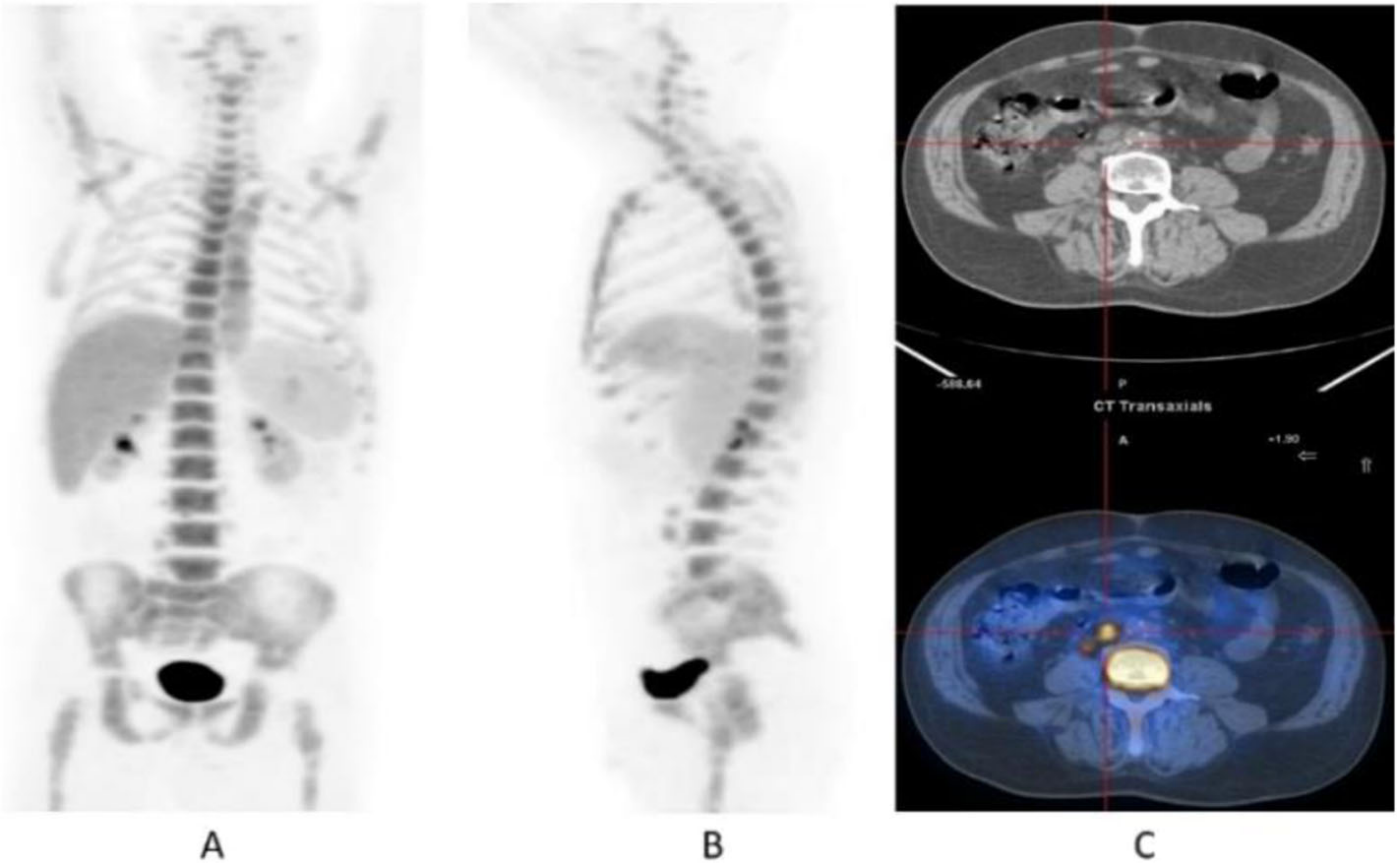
^18^F-FLT, Hodgkin lymphoma, response evaluation. *Clinical history*: 32 y.o. patient with Hodgkin lymphoma after second-line therapy. *PET/CT findings*: moderate uptake of the tracer in two retroperitoneal lymph nodes. a MIP. b MIP in a sagittal view. c CT and fused images
